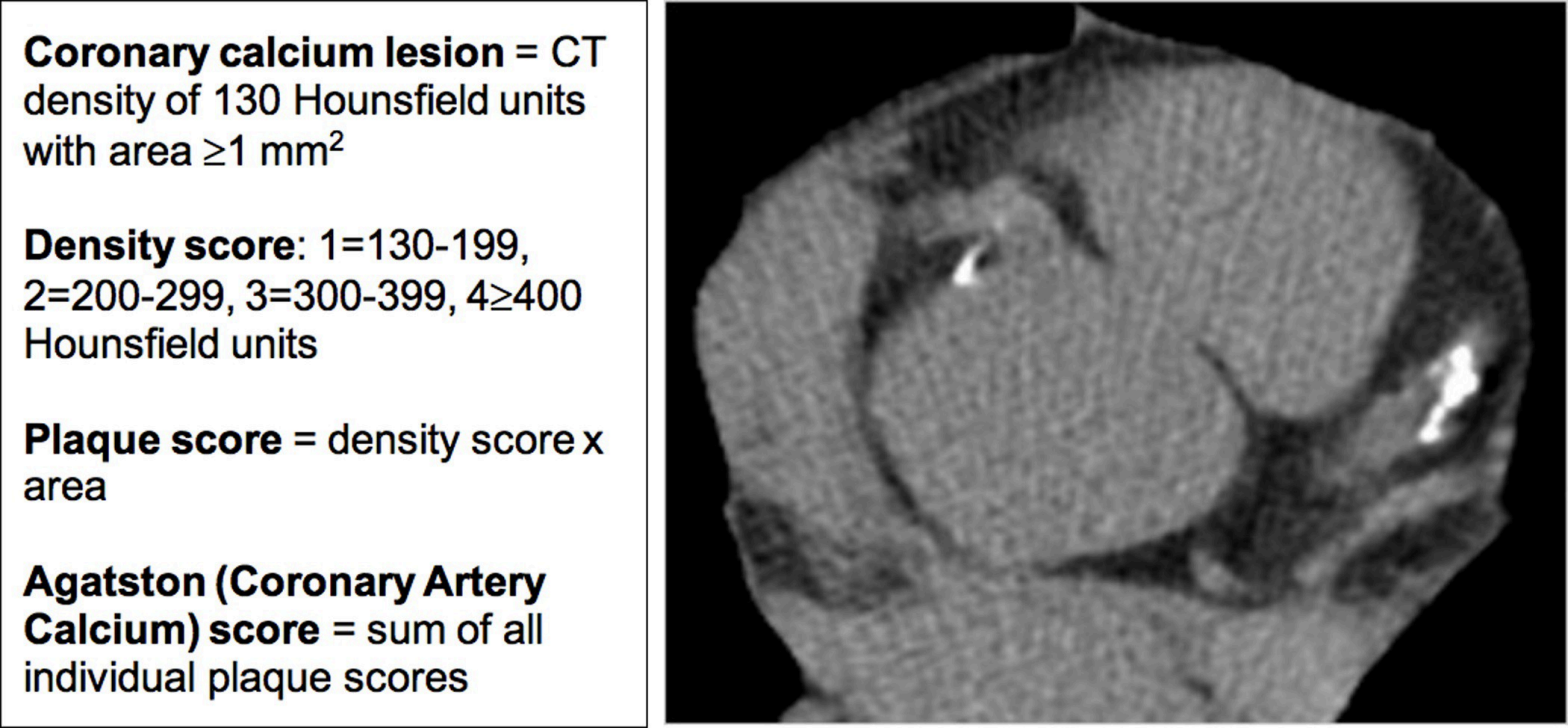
CAC scoring methodology and example image. CAC scoring methodology is depicted on the left. The Agatston (CAC) score is what is used in clinical practice. On the right is an example of a single slice (image) from a CAC scan that demonstrates calcification in the left anterior descending and right coronary arteries. CAC, coronary artery calcium; CT, computed tomography.
